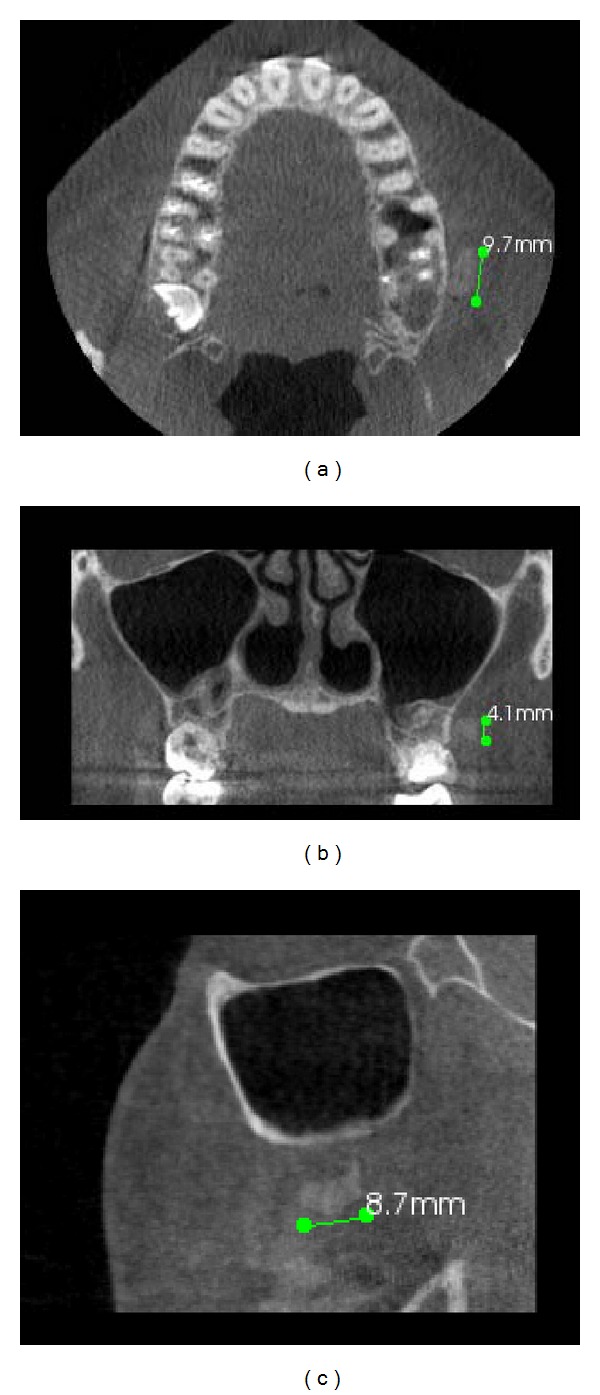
(a) CBCT axial cuts showing the extent of the radio-opacity of the lesion in the vestibular side and then in relationship with the second molar. (b) and (c) Coronal and sagittal CBCT scan image demonstrating also the radio-opacity at the same level of the coronal 1/3 of the root canal. The lesion measured approximately 9.7 × 8.7 × 4.1 mm.
